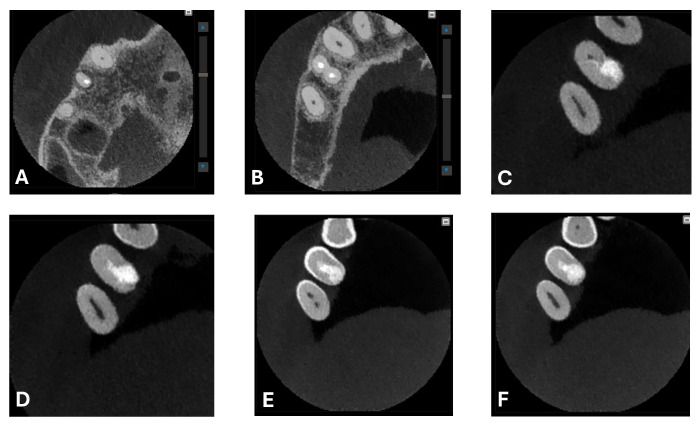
Axial CBCT images of tooth 1.4 after six months of healing. (A–F) Sequential scrolling of the root of tooth 1.4 after six months of healing.
